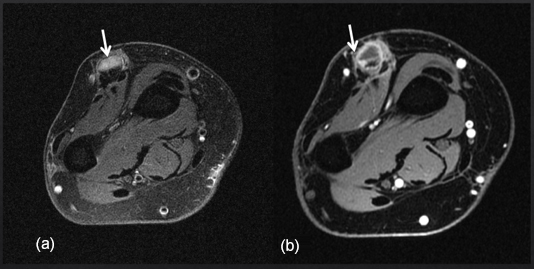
Axial T2-weighted fat-suppressed image (a) and corresponding
